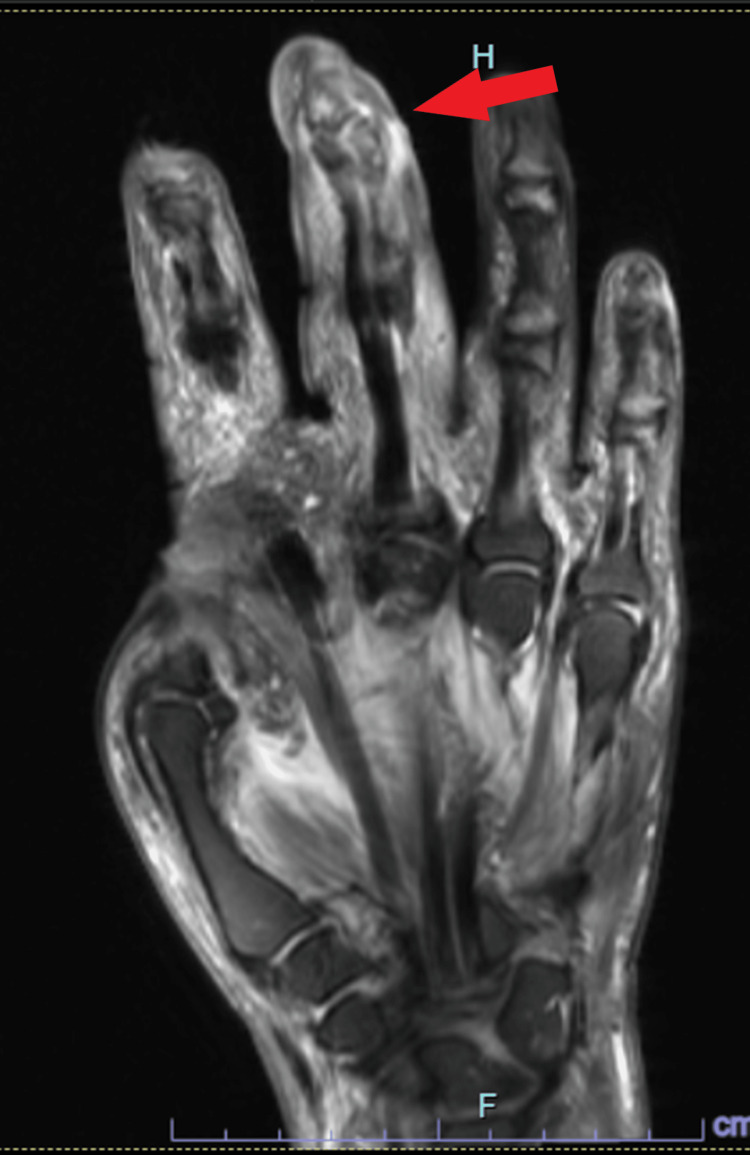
MRI of right hand. Osteomyelitis suspected at about the middle and distal phalanges of the third finger with surrounding cellulitis, myositis with flexor and extensor tenosynovitis.
